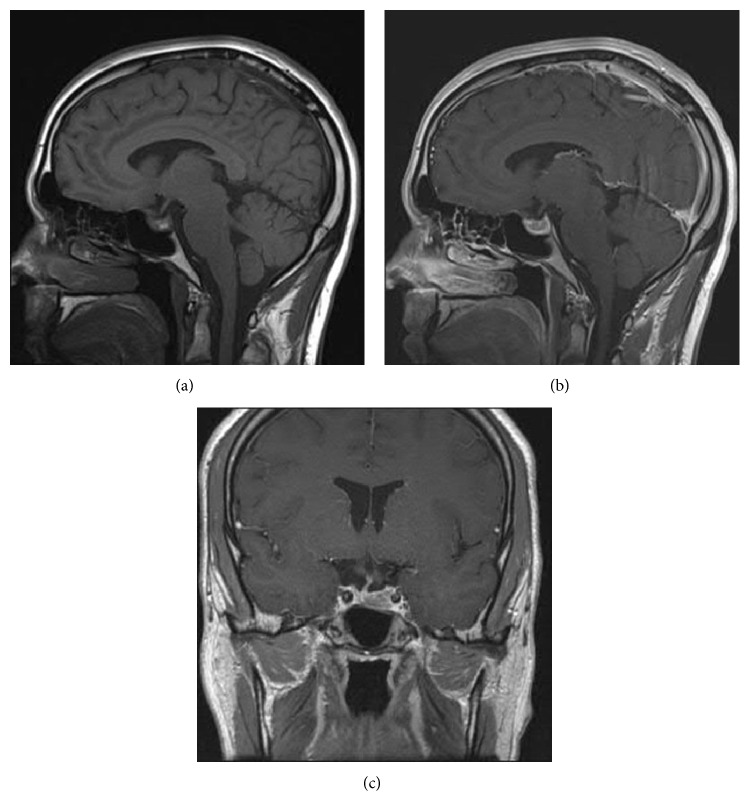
Seven weeks after the MRI in Figure 1, MRI revealed an even smaller sellar lesion on (a) sagittal precontrast T1-weighted, (b) sagittal postcontrast T1-weighted, and (c) coronal T1-weighted images. The enhancement and heterogeneity of the pituitary mass can still be seen, although less than in Figure 2. The optic chiasm is even more visible in the coronal T1-weighted image with the pitutitary mass smaller in vertical length. The pituitary stalk can still be seen deviated to the right.
